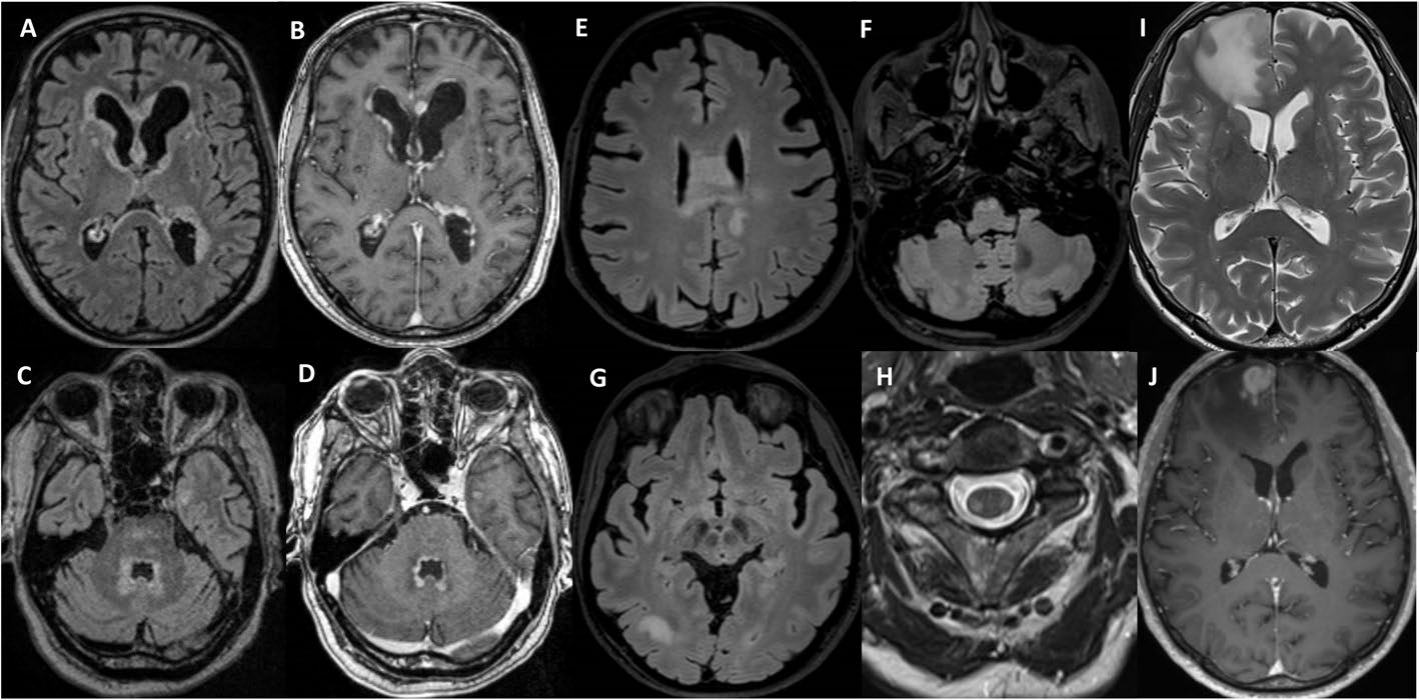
Brain MRI of the three cases. Case 1: Initial MRI with periventricular T2/FLAIR hyperintensities (A, C) and nodular gadolinium enhancement (B, D). Case 2: Brain MRI with T2/FLAIR hyperintensities of the corpus callosum extending to the corona radiata (E), of the right parieto-occipital subcortical white matter (G) and of the bilateral pyramids (F). Spinal MRI with bilateral lateral columns T2 hyperintensities (H). Case 3: Brain MRI with T2 hyperintense fronto-sagittal lesion and perilesional edema (I) and strong gadolinium enhancement (J)
